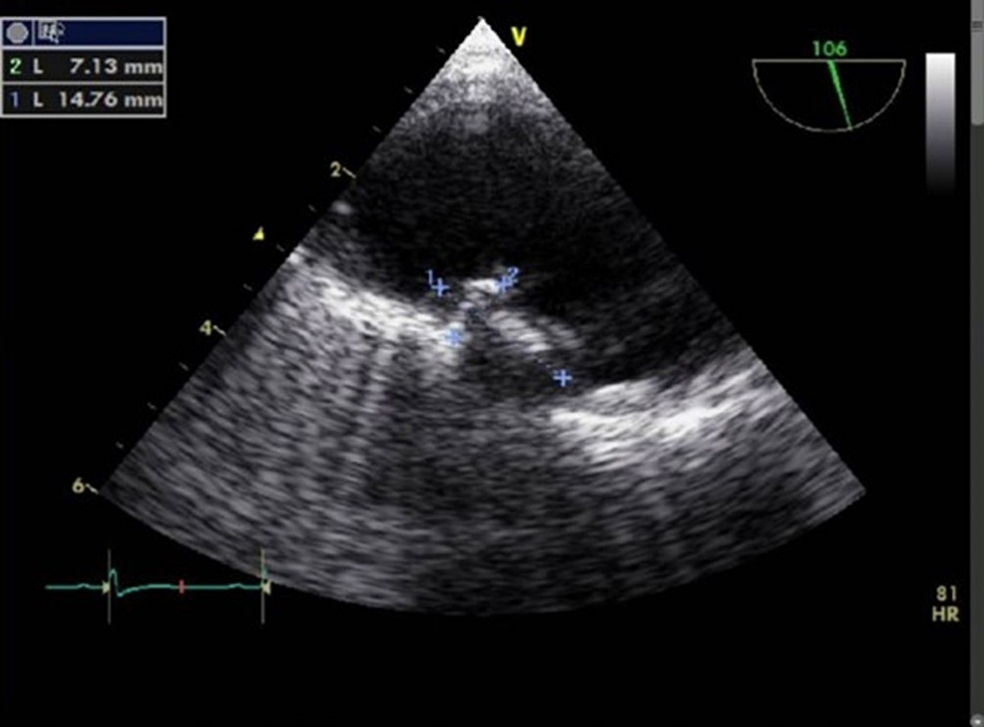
Thrombus in the aortic arch seen on transesophageal echocardiography.
